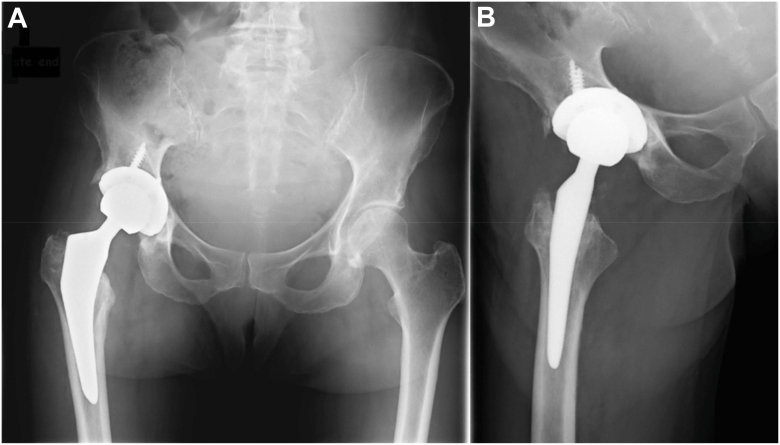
X-rays at the follow-up appointment in June 2024 showing no evidence of loosening or fracture of the right hip prosthesis. (a) Anterior-posterior pelvis view. (b) Axial view of the right hip according to Lauenstein.
